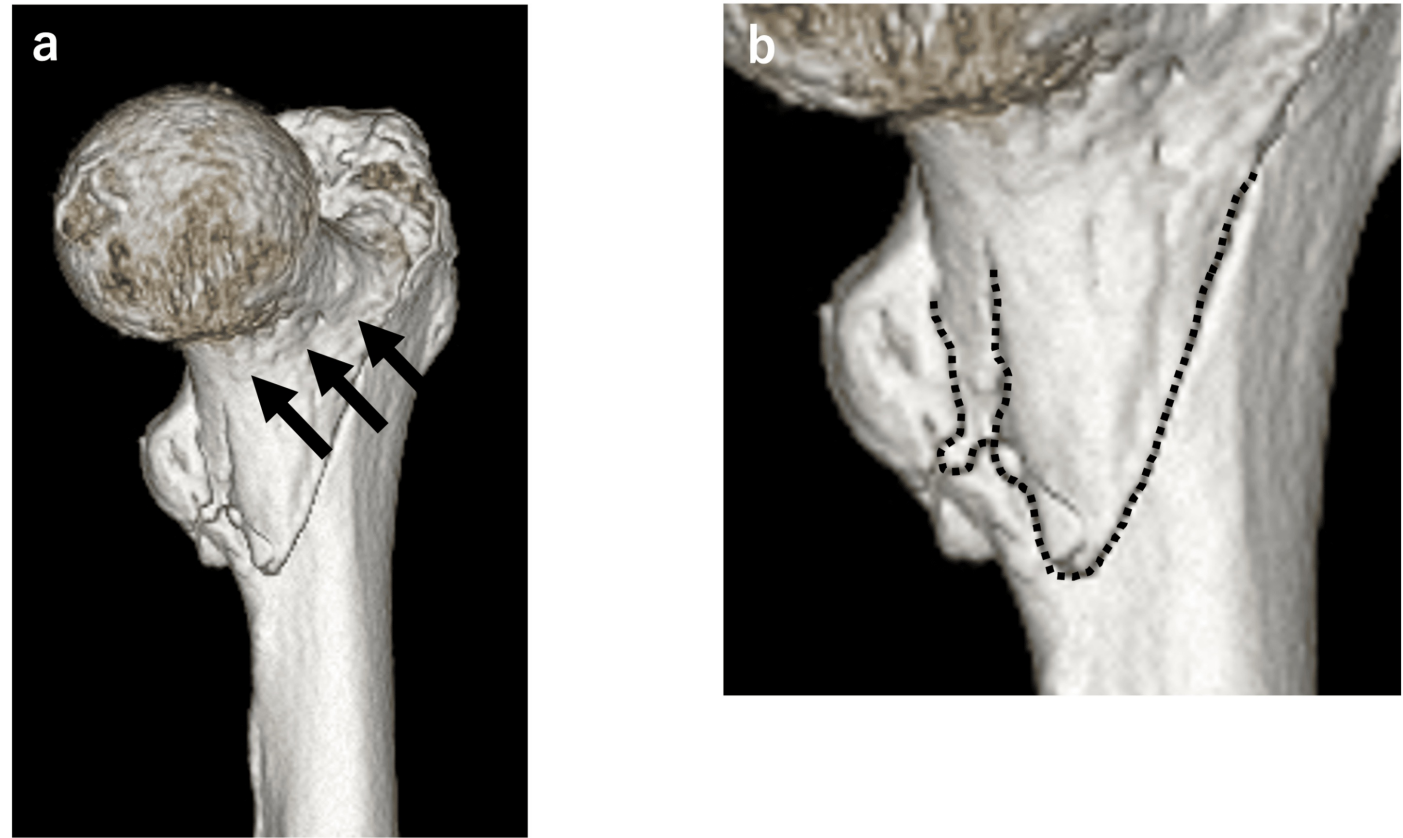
Pre-operative 3D CT images. (a) The fracture line from calca to the proximal direction appears to extend horizontally at the level of the neck (black arrows). (b) "Butt" sign (black dotted line).
